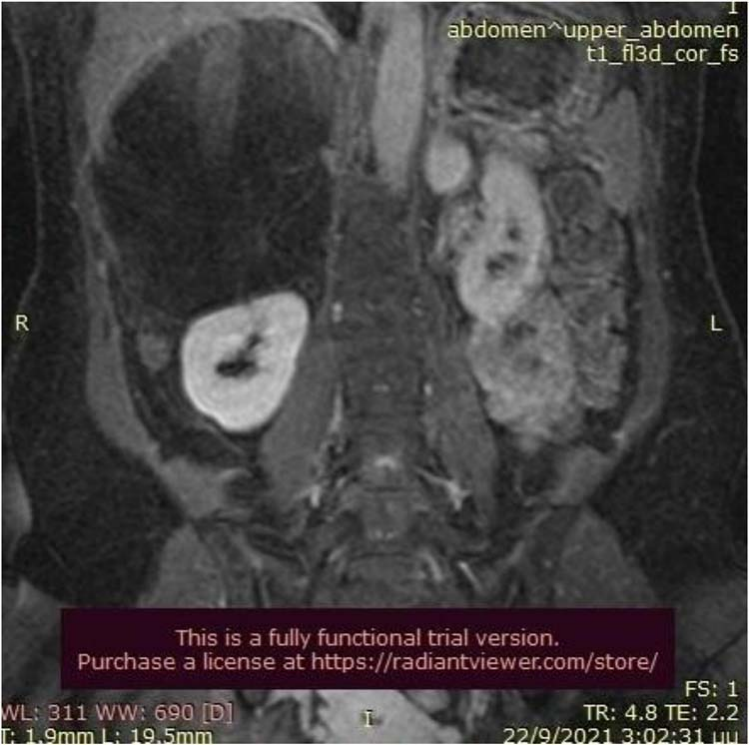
Abdominal MRI scan (frontal plane).
